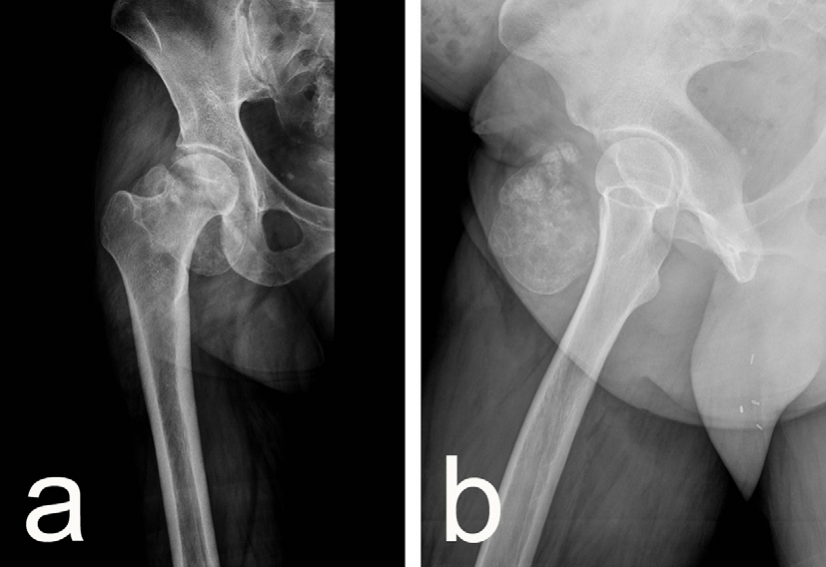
(a, b) Preoperative radiographs showed a well-circumscribed ovoid radiopaque mass.
